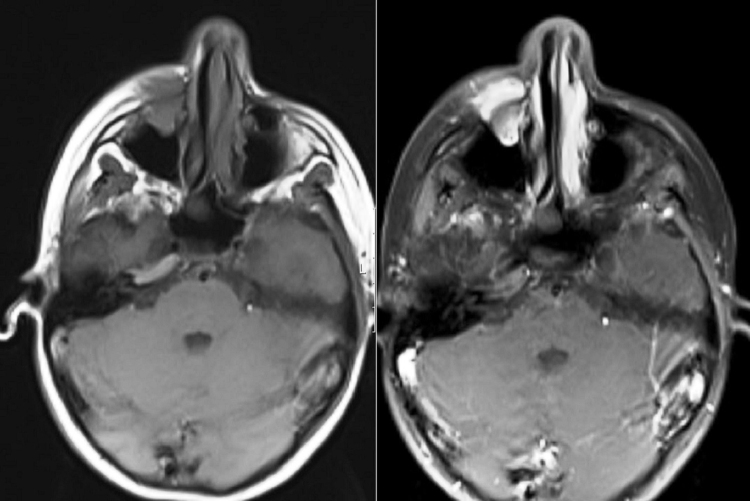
A axial MRI of the sinuses revealed a mass in the right maxillary sinus.
